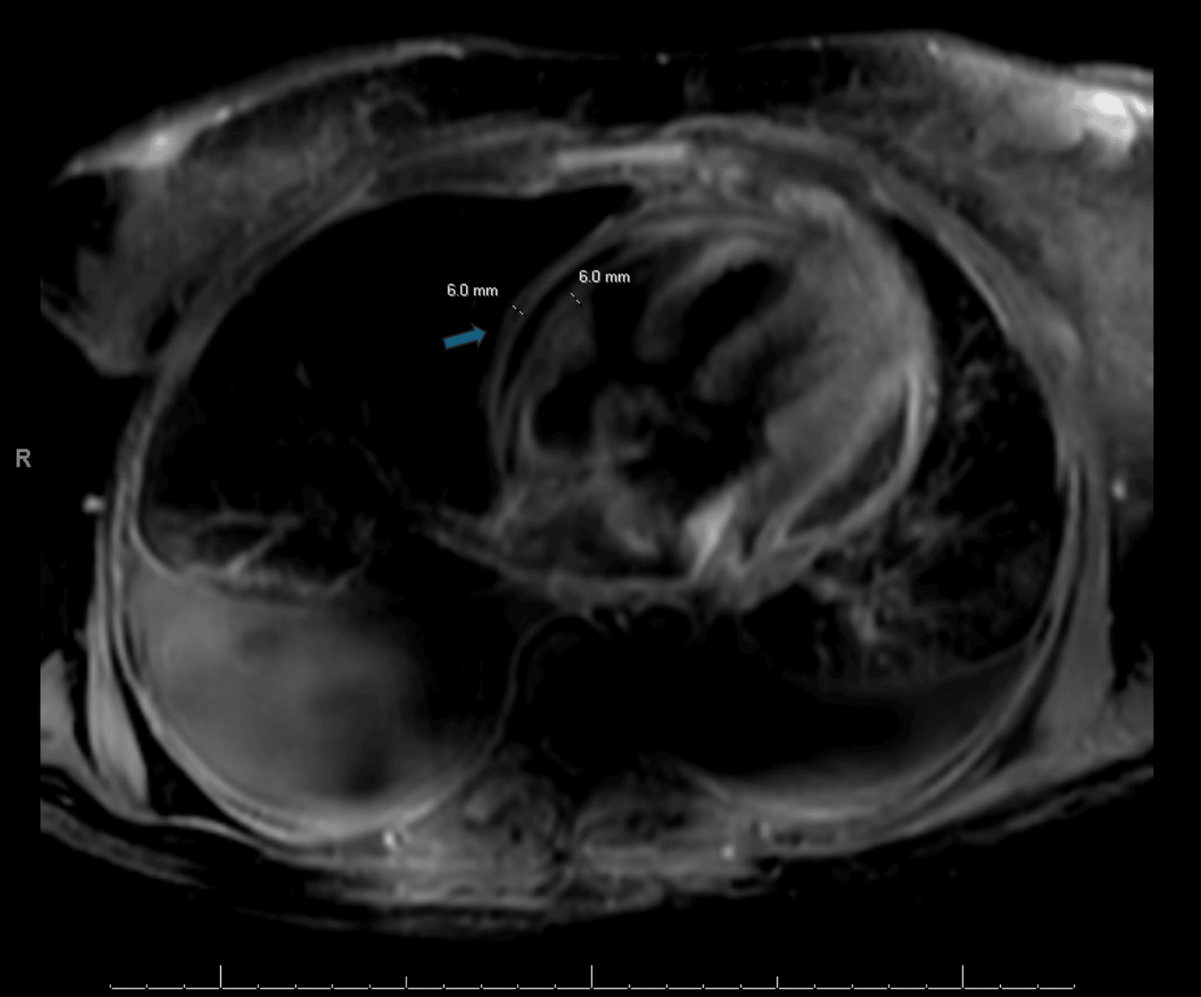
Cardiac magnetic resonance imaging (MRI) of the heart (four-chamber view) The image demonstrates pericardial thickening, indicated by the blue arrow. In constrictive pericarditis, pericardial thickening reflects inflammation and fibrosis, resulting in a rigid and inelastic pericardium. This rigidity restricts diastolic filling, limiting the heart’s capacity to expand during relaxation. Consequently, filling pressures rise, which aligns with the clinical presentation of constrictive pericarditis.
